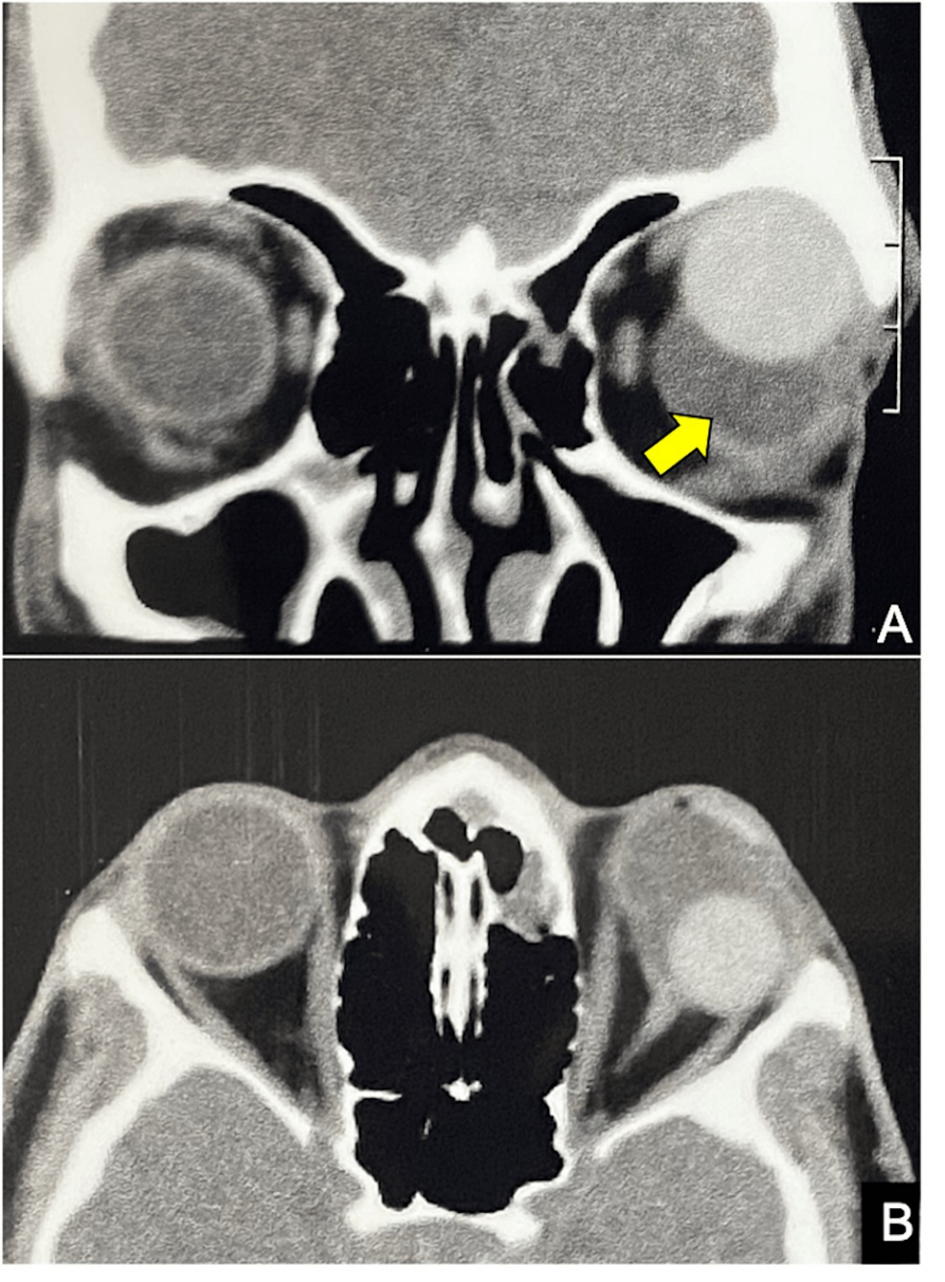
Plain CT scan orbits CT scan image with coronal and sagittal cuts demonstrating the location of the cyst (yellow arrow)
